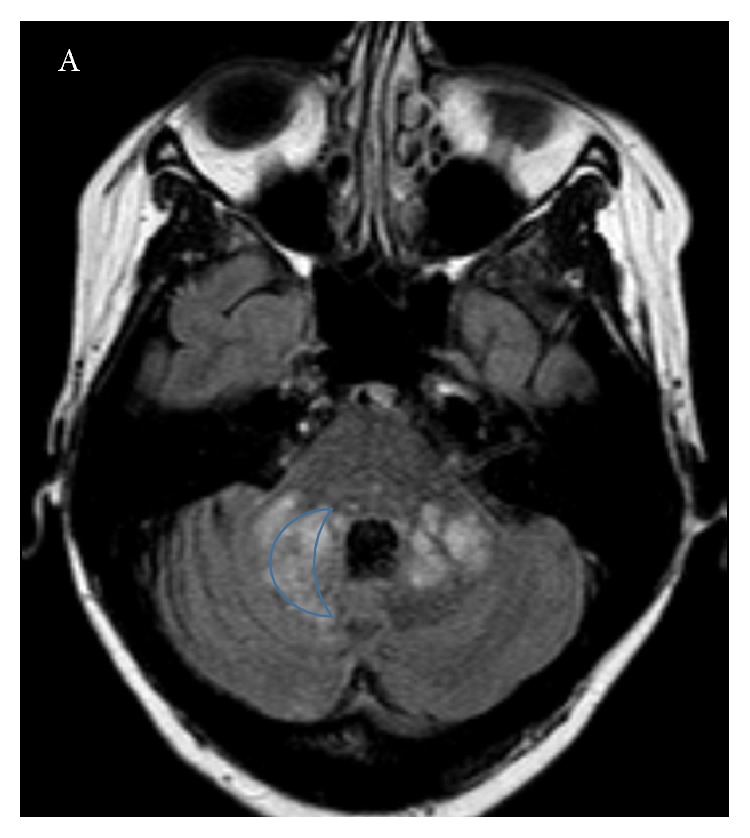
45-year-old woman with a 10-year history of RRMS was started on natalizumab. She did well for six years and then developed gait abnormality and fatigue. MR imaging demonstrates large lesions in the cerebellar peduncles demonstrating a “crescent” shape.
